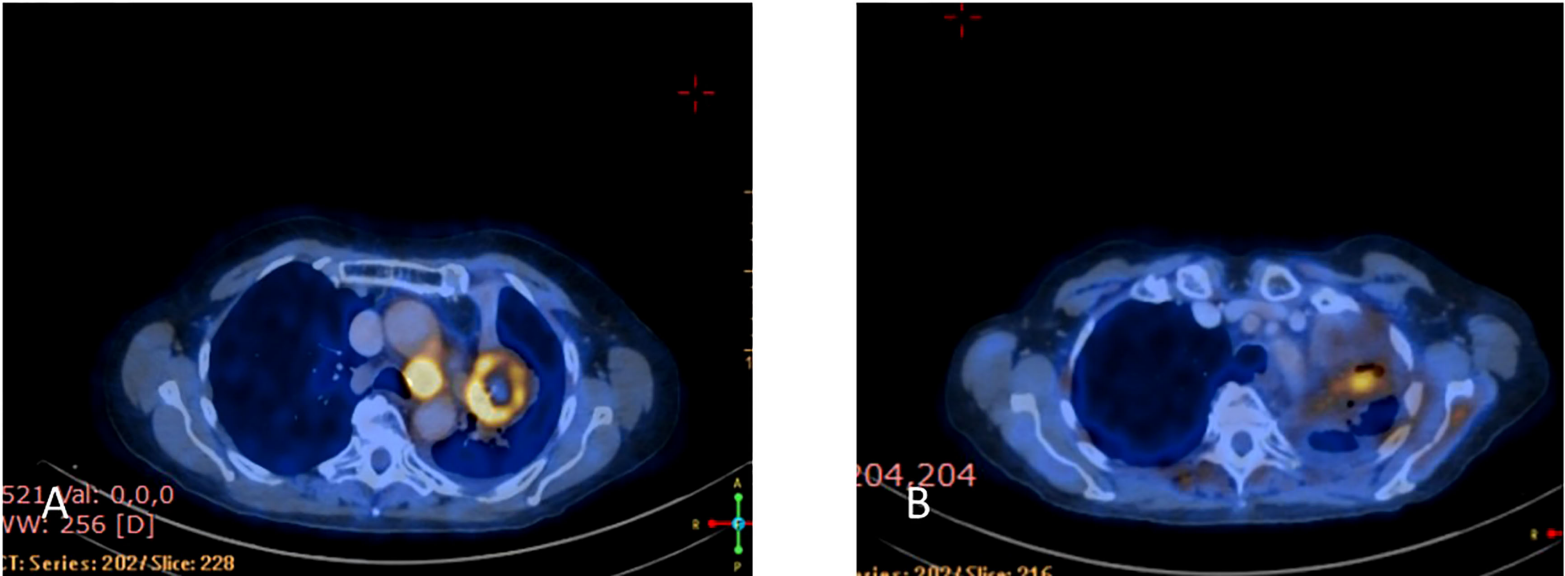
(A) Coronal PET-CT scan from October 2021 shows a left lung mass and ipsilateral pleural effusion with increased uptake of FDG-18, indicating disease progression on Osimertinib. (B) Three months later, January 2022, showed significantly decreased uptake of FDG-18, indicating partial response following adding Amivantamb to Osimertinib.
